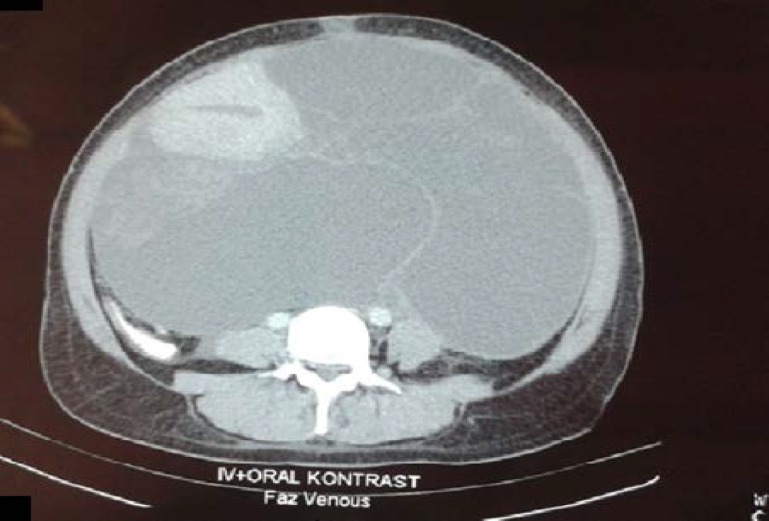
Computered Tomography (CT) of the giant retroperitoneal leiomyoma with cystic degeneration and a normal uterus
